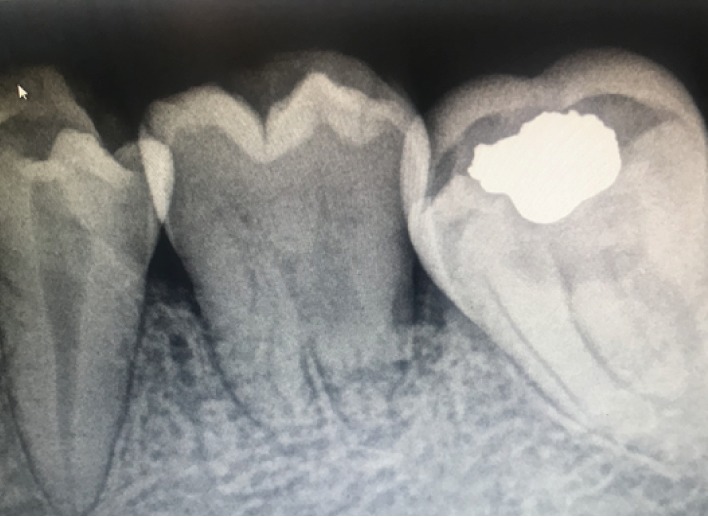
3-year periapical follow-up radiograph.
